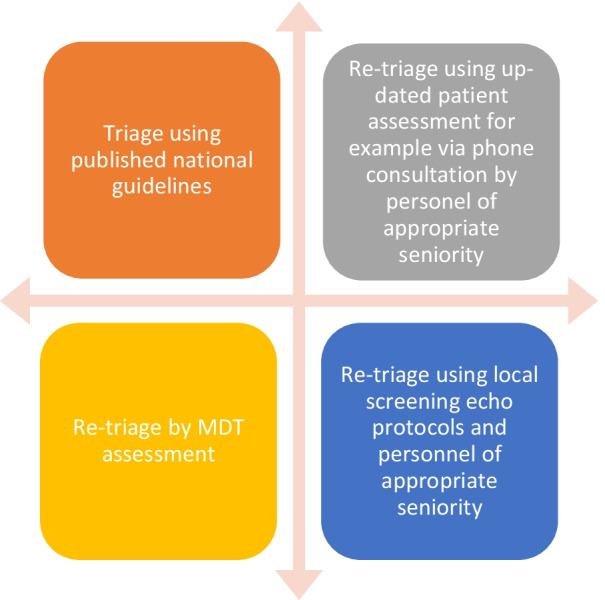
Model for the effective triage of existing (long-standing) and new transthoracic echocardiography requests by appropriately trained clinical specialists only and in-sight of the patients medical records
